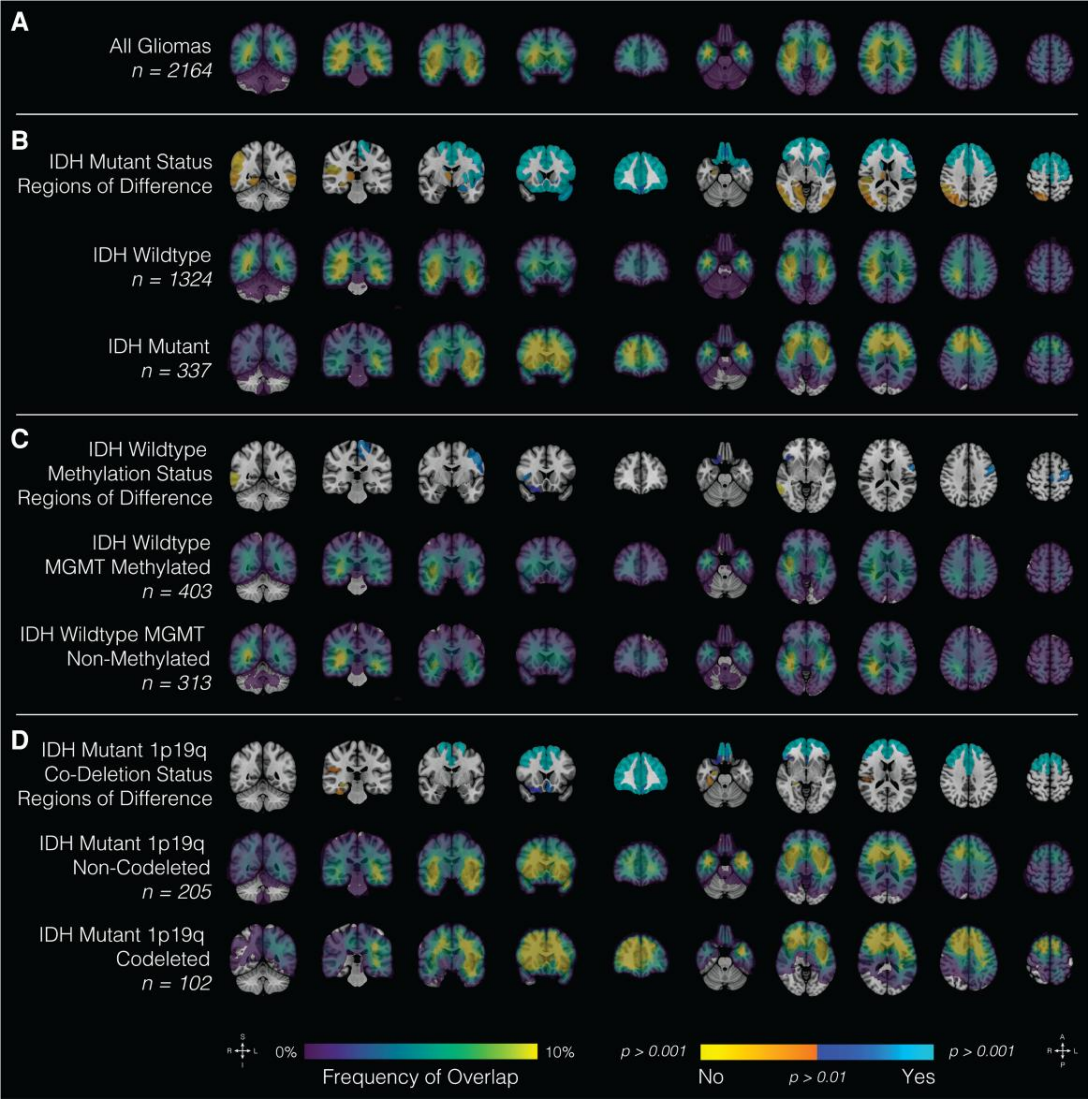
Glioma type and location. Probabilistic maps showing spatial distribution of all gliomas (A) and by molecular subtypes: (B) IDH-mutant status, (C) IDH-wild-type methylation status and (D) IDH-mutant 1p19q co-deletion status. Results are overlaid on the coronal (left half of the image) and axial (right half of the image) T1-weighted MNI brain. Scales indicate *t*-values (first row) and frequency of tumour overlap in percentages (remainder of the rows). IDH, isocitrate dehydrogenase; MNI, Montreal Neurological Institute; T1-weighted, T1-weighted magnetic resonance imaging; 1p19q, co-deletion of chromosome arms 1p and 19q.
